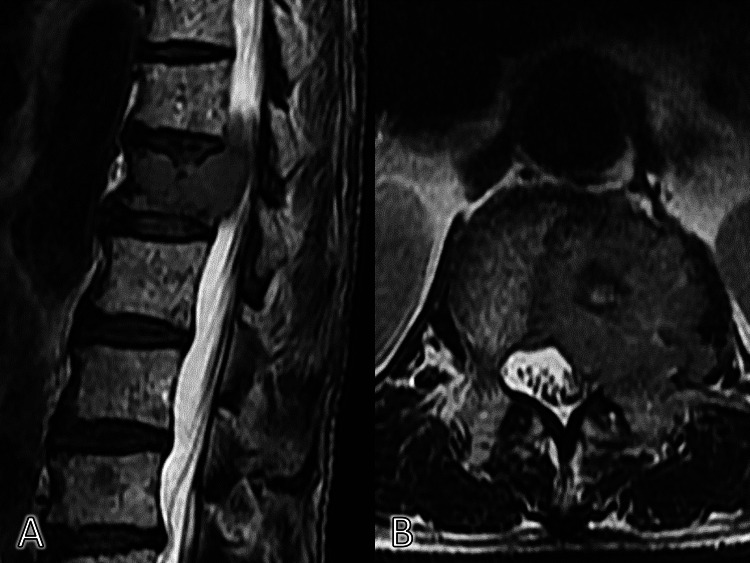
MRI of the vertebrae (A. Sagittal plane, B. Coronal plane). The osteolytic lesion in the first lumbar vertebra invading the left trabeculae on MRI.
